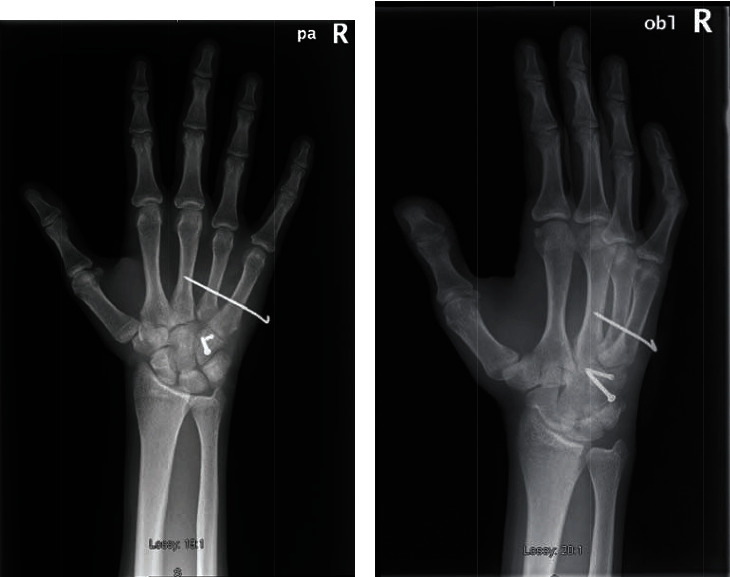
(a, b) X-ray right hand PA and oblique 4 weeks postoperatively demonstrating satisfactory position, alignment, and evidence of healing.
